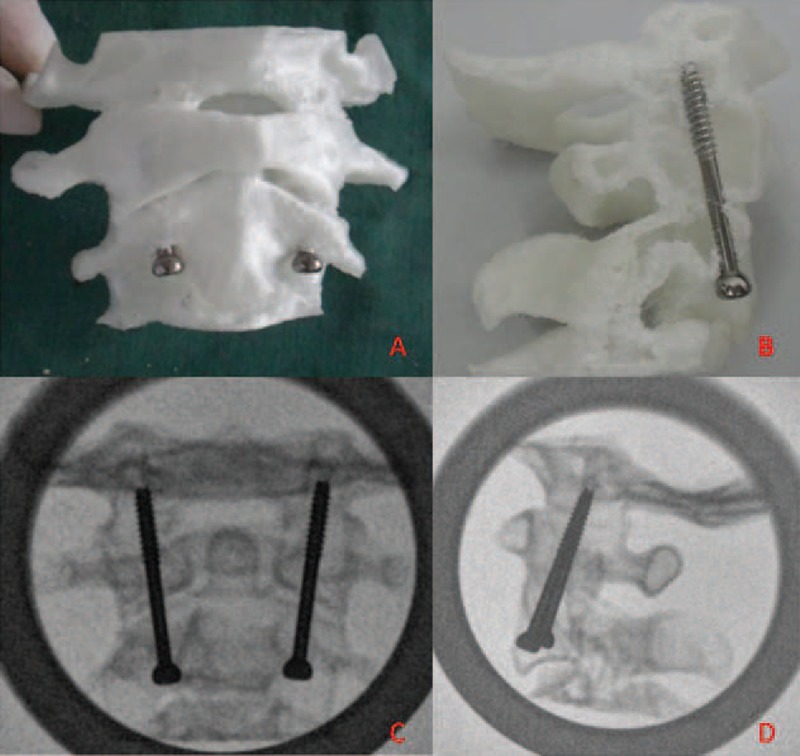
The photo of the 3D printed models after the anterior occiput-to-axis screws were introduced (A and B), the AP (C) and lateral (D) X-ray films of the 3D printed models after the anterior occiput-to-axis screws were introduced.
